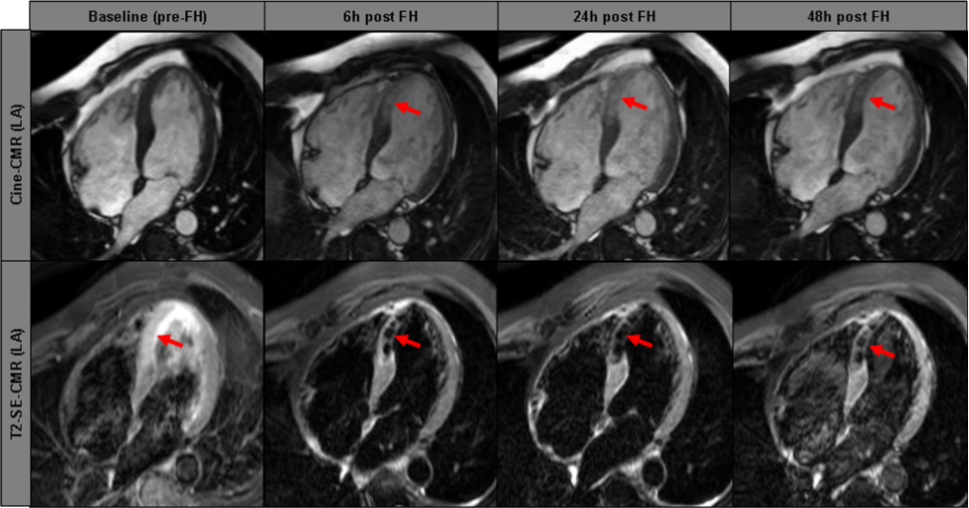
Exemplary cardiovascular magnetic resonance images in the long-axis view of a study patient with a septal myocardial infarction who underwent pre-Feraheme^™^ (FH) (baseline) and post-Feraheme^™^ (after 6, 24, 48 h) cardiovascular magnetic resonance imaging studies, respectively. The first line shows cine-cardiovascular magnetic resonance images at different time points with proof of hyperenhancement in the septal wall at 6–48 h post-Feraheme^™^ (red arrows). The second line shows T2-weighted short-tau inversion recovery-spin echo images at different time points with proof of hypoenhancement in the septal wall at 6–48 h post-Feraheme^™^ (red arrows). Reproduced with permission from Yilmaz et al. [57]
